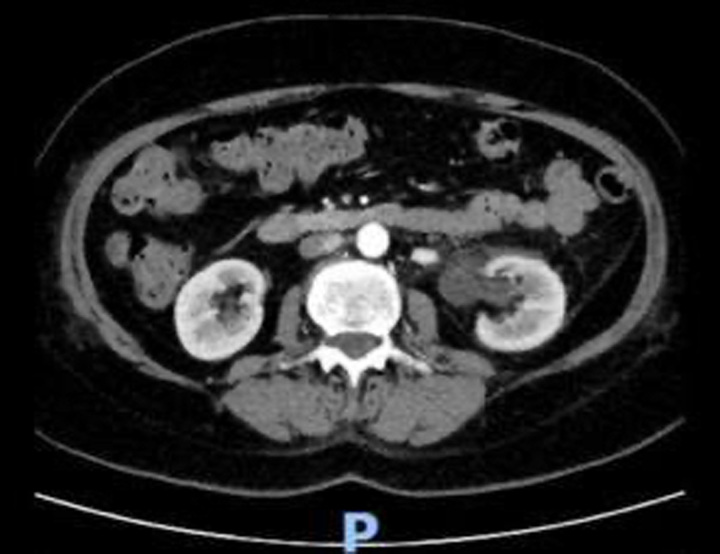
Computed tomography abdomen and pelvis pre-IV and post-IV contrast showed moderate left hydroureteronephrosis with perinephric/periuretericfat stranding and pocket of free fluid within the left anterior pararenal space.
